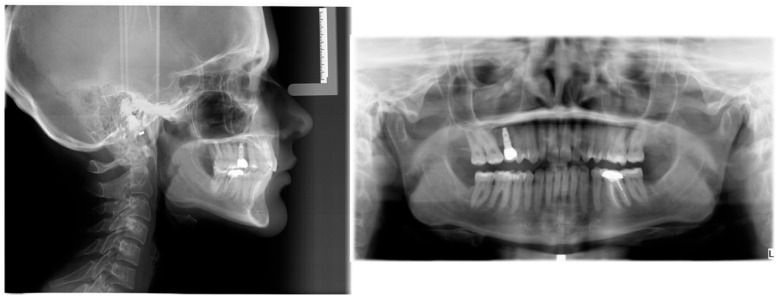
Pre-treatment lateral cephalometric radiograph taken in habitual occlusion, panoramic radiograph taken with a standard bite block technique.
